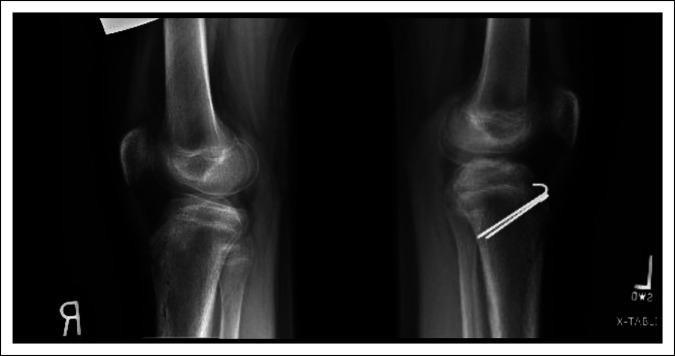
Radiograph of the right knee reveals healing of the tibial tubercle fracture and that of the left knee reveals appropriate positioning of Kirschner wires with healing of the tibial tubercle fracture. Proximal and distal tibial tunnels can be appreciated in the right knee.
